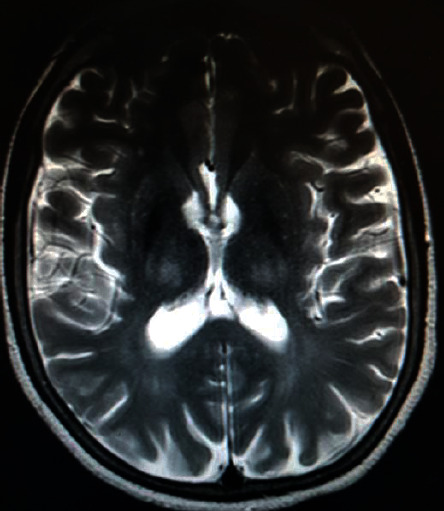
Axial T_2_-weighted MRI image showing increased signal intensity in basal ganglia and thalamus (case-1).
